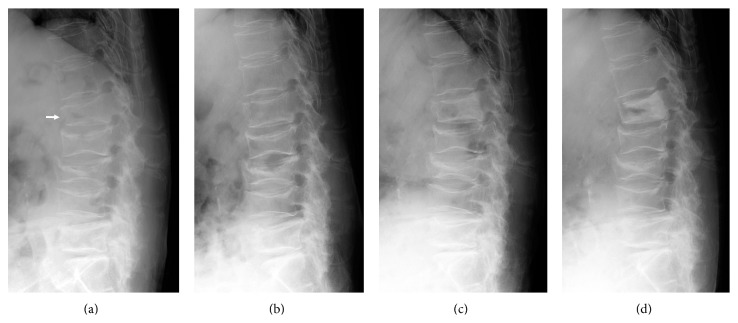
Plain lateral X-rays of the lumbar spine. (a) At the initial visit, the L1 vertebra shows a wedge-shaped deformity with a vertebral kyphosis angle (VKA) of 20° and a small intravertebral cleft (arrow). (b) At 2 weeks after injury, VKA of L1 is decreased (6°) compared to baseline, showing unstable vertebra. (c) At 2 months after injury, VKA of L1 is 8° with consolidation of the posterior wall. (d) At 7 months after injury, VKA of L1 is unchanged (8°) in the corrected position, although anterior intravertebral cleft remains.
